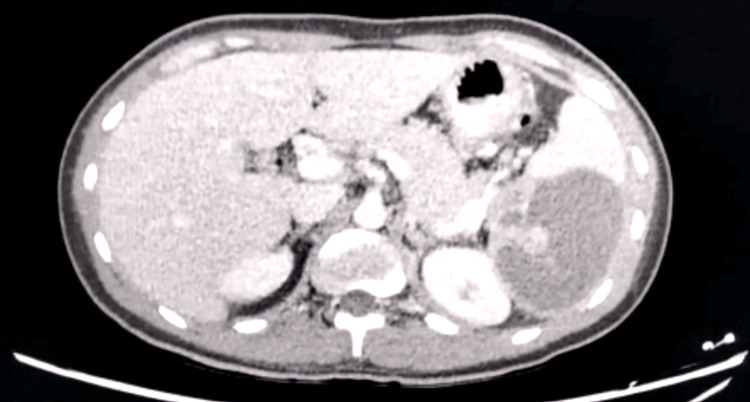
Axial view of the computed tomography (CT) abdomen showing a uniloculated hypodense lesion at the lower to mid-pole of the spleen measuring 10 x 12 cm
